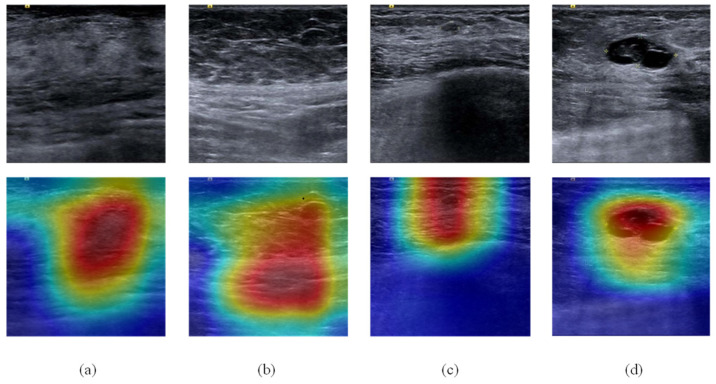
Heat map of the classification network for different ultrasound images. (a,b) are images without lesion, (c,d) are images with lesion.
